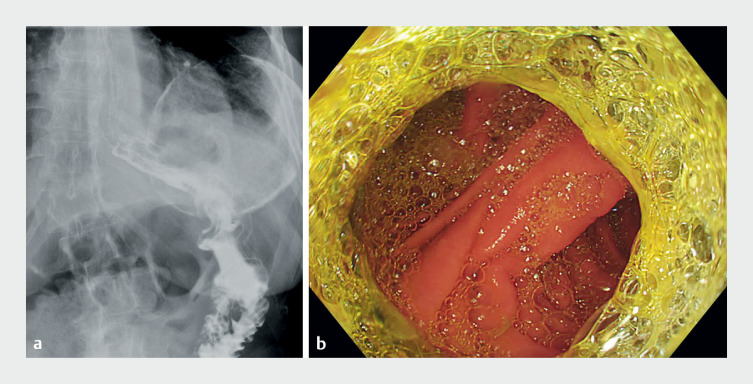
Endoscopic and radiological imaging 3 months after endoscopic ultrasound-guided gastroenterostomy.
